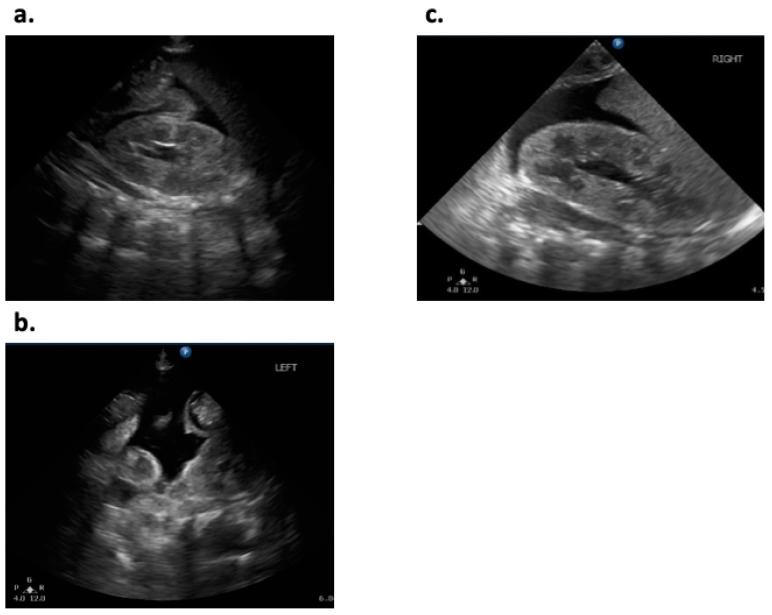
Abdominal ultrasonography of the case. Ascites was noted on (a,b) day 85 and (c) day 98.
